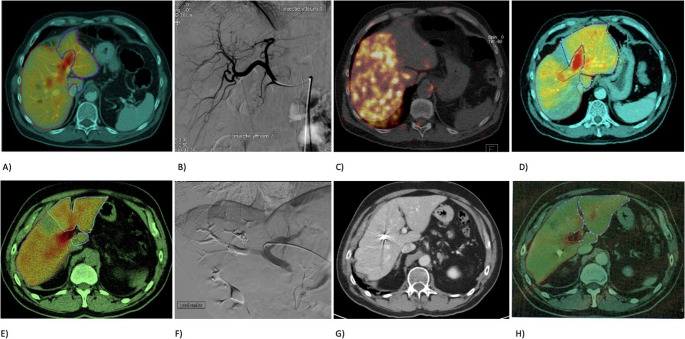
Pictorial overview demonstrating RL (A–D) and PVE (E–H). RL: A patient with low baseline FLR function (1.2%/min/m^2^) on HBS. B Scout angiography with intra-arterial ^99m^Tc-MAA injection; SPECT-CT shows accurate right-lobe targeting without extrahepatic targeting. C RL performed according to MAA injection; Y90 PET-CT confirms accurate delivery. D Two months later, HBS shows adequate FLR hypertrophy and function (3.0%/min/m^2^). PVE: E patient with baseline FLR function of 2.4%/min/m^2^. F PVE of segments 5–8 using particles, coils, and Amplatzer plug. G CT after one month demonstrates hypertrophy of segments 1–4. H HBS confirms sufficient FLR function (3.6%/min/m^2^)
